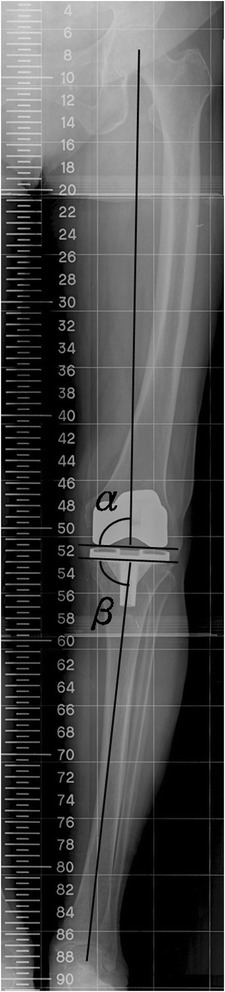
Radiograph showing the measurement of component alignment angles in the coronal plane. The alignment of the femoral components was measured by the intersection of a *line* drawn across the base of the femoral component and the mechanical axis. The alignment of the tibial components was measured by the intersection of a *line* drawn across the base of tibial component and the mechanical axis. Radiographic evaluation system originally appeared in J Bone Joint Surg Am. 2009;91:14–19. *α* frontal femoral angle, *β* frontal tibial angle
